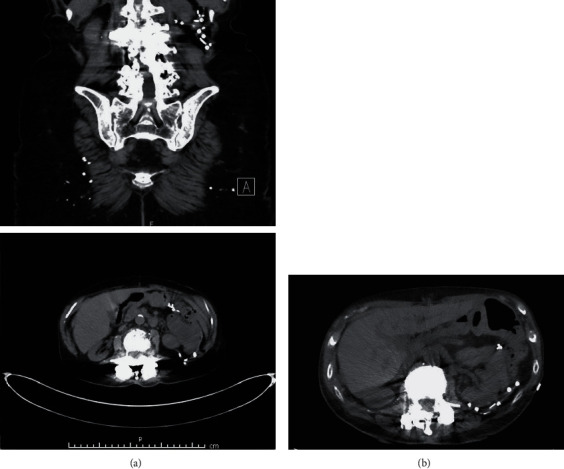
(a) Preoperative CT scan demonstrating a superior lumbar hernia defect containing retroperitoneal fat. (b) Postoperative imaging 3 months after surgery.
